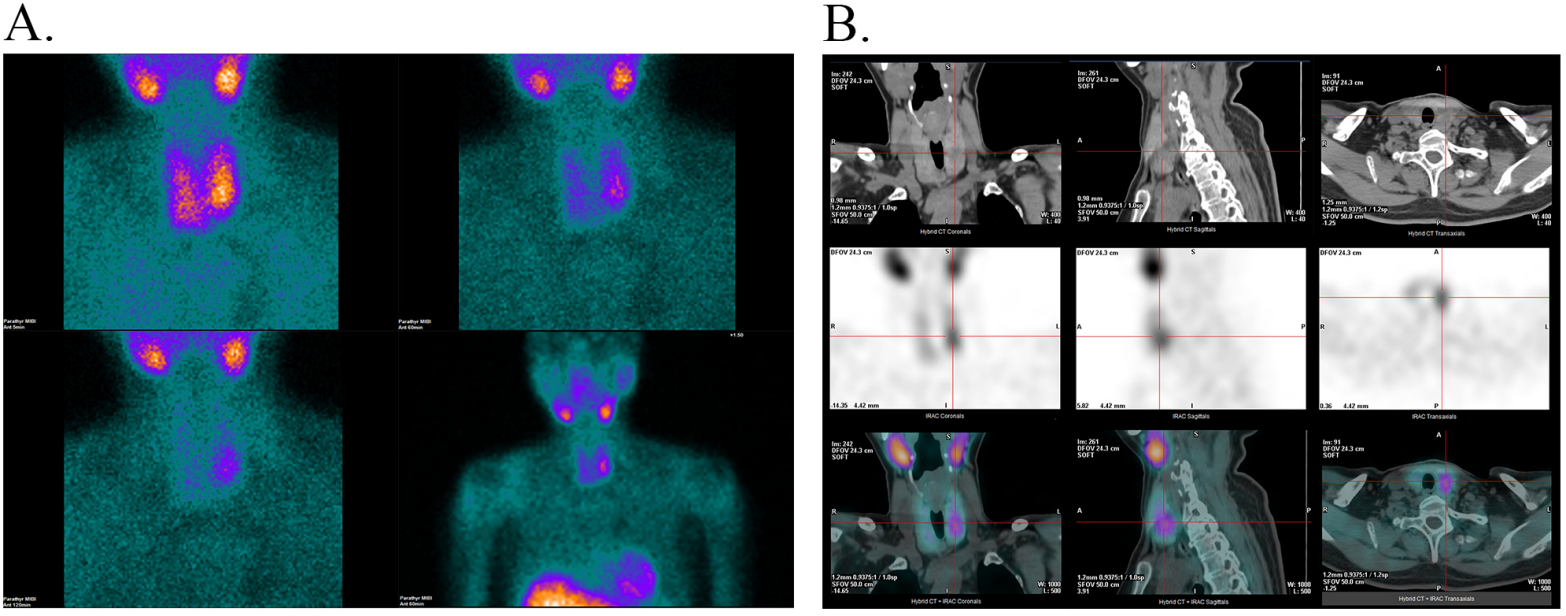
(A) 5 minutes, 1 hour and 2 hours after ^99m^Tc-MIBI injection. (B) The ^99m^Tc-MIBI scintigraphy with CT fusion imaging findings.
